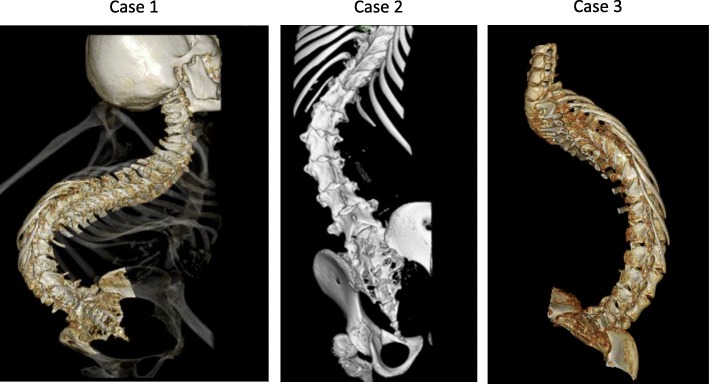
Reconstructed three-dimensional CT images of three cases. The volume-rendering reconstructions show marked scoliosis
